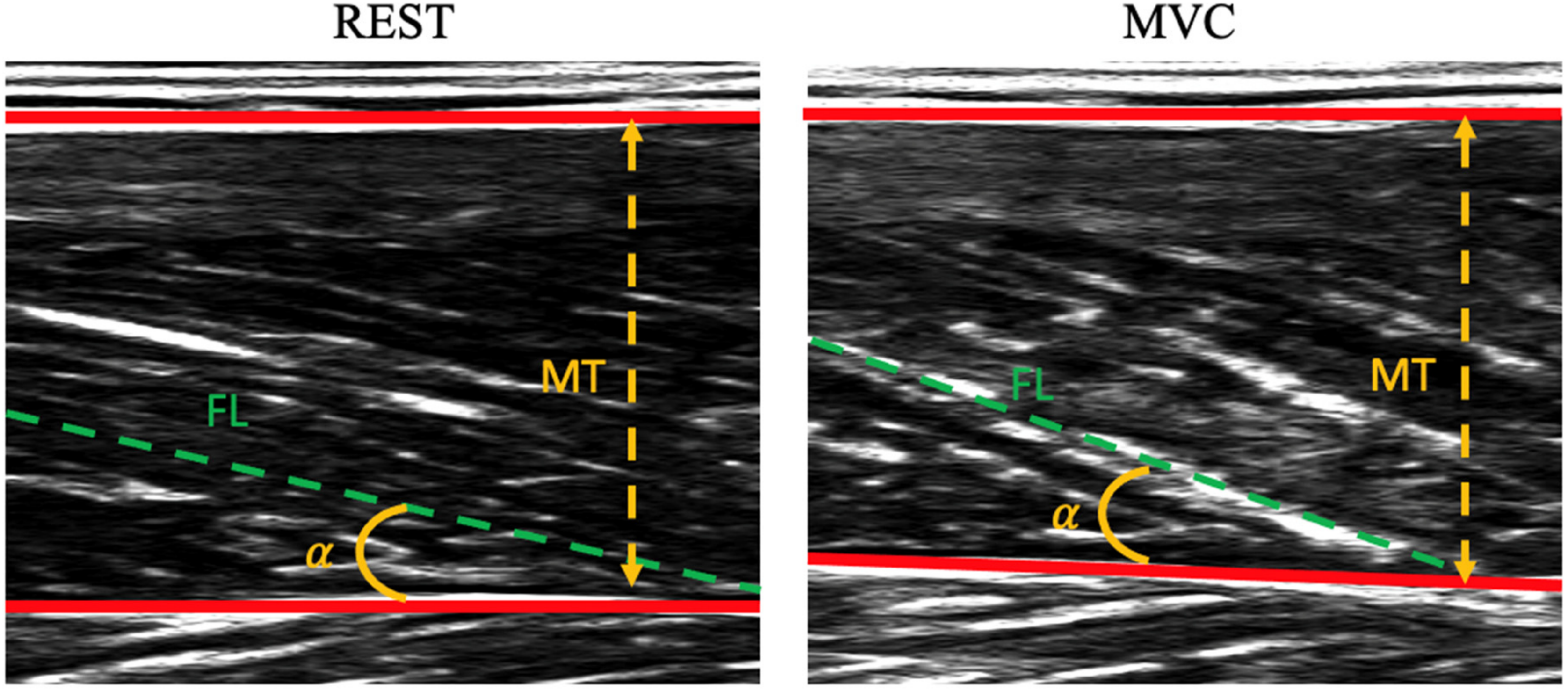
Representative ultrasound images of vastus lateralis at rest (panel on the left) and during contraction (panel on the right). Data refer to a patient with Parkinson's disease. MT, muscle thickness; *α*, pennation angle (PA); FL, fascicle length.
